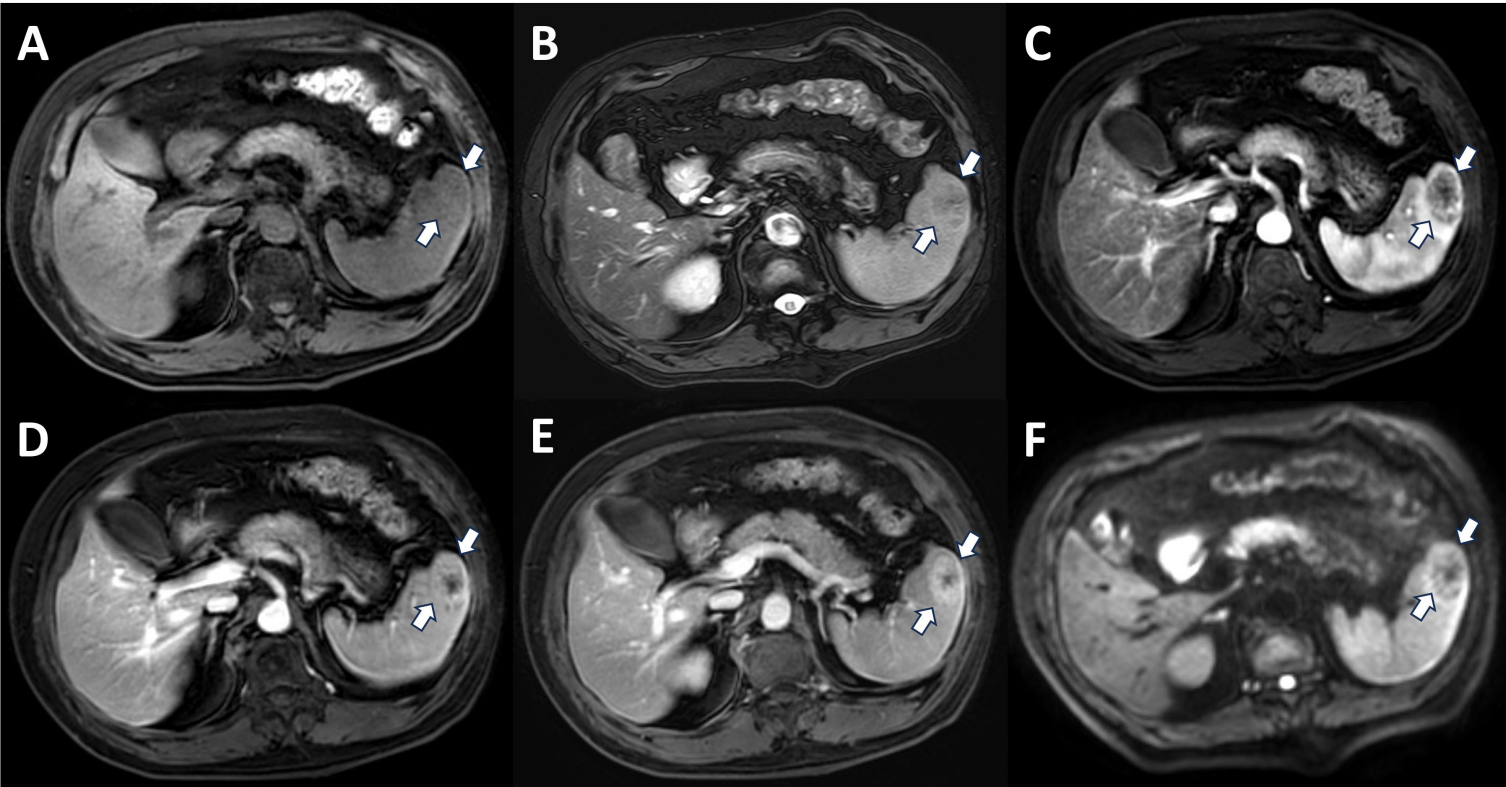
MRI of the patient’s spleen. T1WI showed a mass of fuzzy low-signal shadows on the upper pole of the spleen (A). The lesion site on T2WI was clearer, showing a slightly oval low-signal shadow (B). On enhanced MRI, the edge of the lesion was enhanced, and the punctate enhancement was obvious. Irregular patchy low-signal shadows were observed in the center of the lesion, and delayed patchy enhancement was observed inside the lesion in the portal phase and delayed phase (C-E). On DWI, the lesion site showed a spoke wheel pattern sign of low signal in the center, and the edge showed irregular punctate enhancement (F). MRI, magnetic resonance imaging; T1WI, T1 weighted image; T2WI, T2 weighted image; DWI, diffusion weighted imaging.
